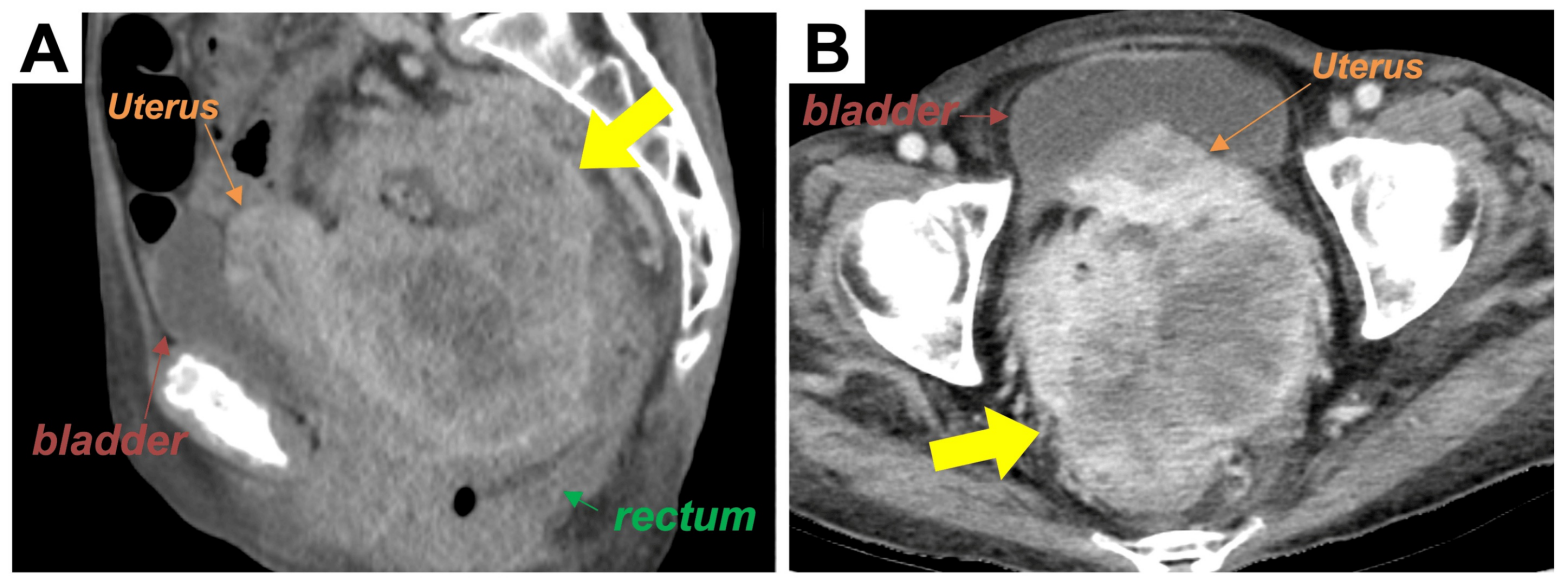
Baseline computed tomography images of the primary tumor (A) Sagittal plane. (B) Axial plane. Yellow arrows indicate the tumor.
